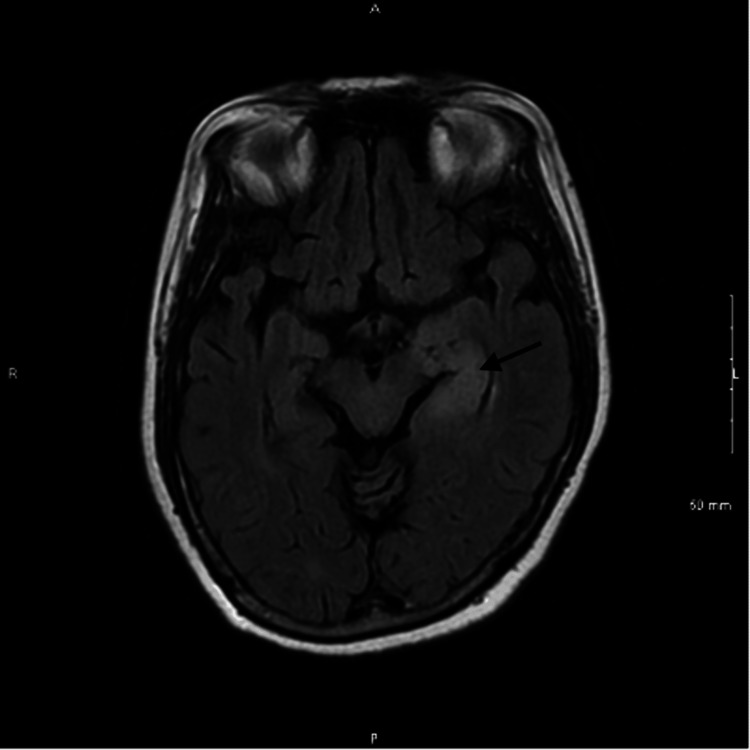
This axial view of brain MRI FLAIR with no contrast shows an increased T2 signal involving the medial left temporal lobe, which is consistent with limbic encephalitis. MRI: magnetic resonance imaging; FLAIR: fluid-attenuated inversion recovery
